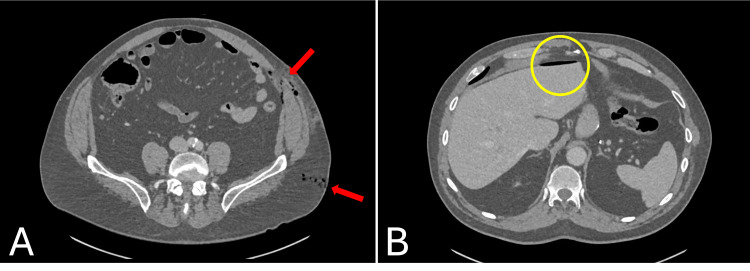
CT scan demonstrating free gas locules in subcutaneous, preperitoneal, and intraperitoneal space (as indicated by red arrows in image A) and free gas above the liver (as indicated by yellow circle in image B) CT: computed tomography
